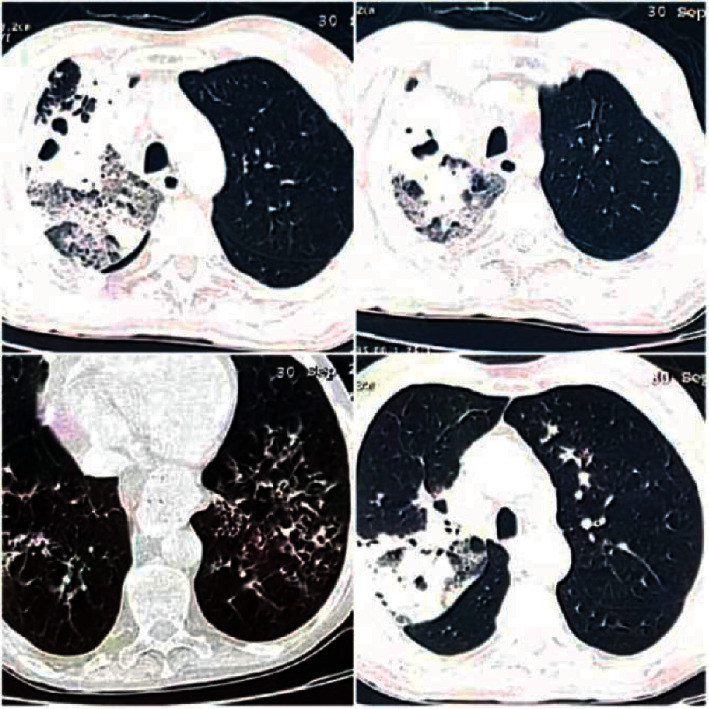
CT scan images showing foci of pulmonary condensation and cavitations of the right upper lobe with diffused ground-glass opacities and multiple bilateral foci of nodules and micronodules suggesting CO-RADS 5 with signs of active tuberculosis.
